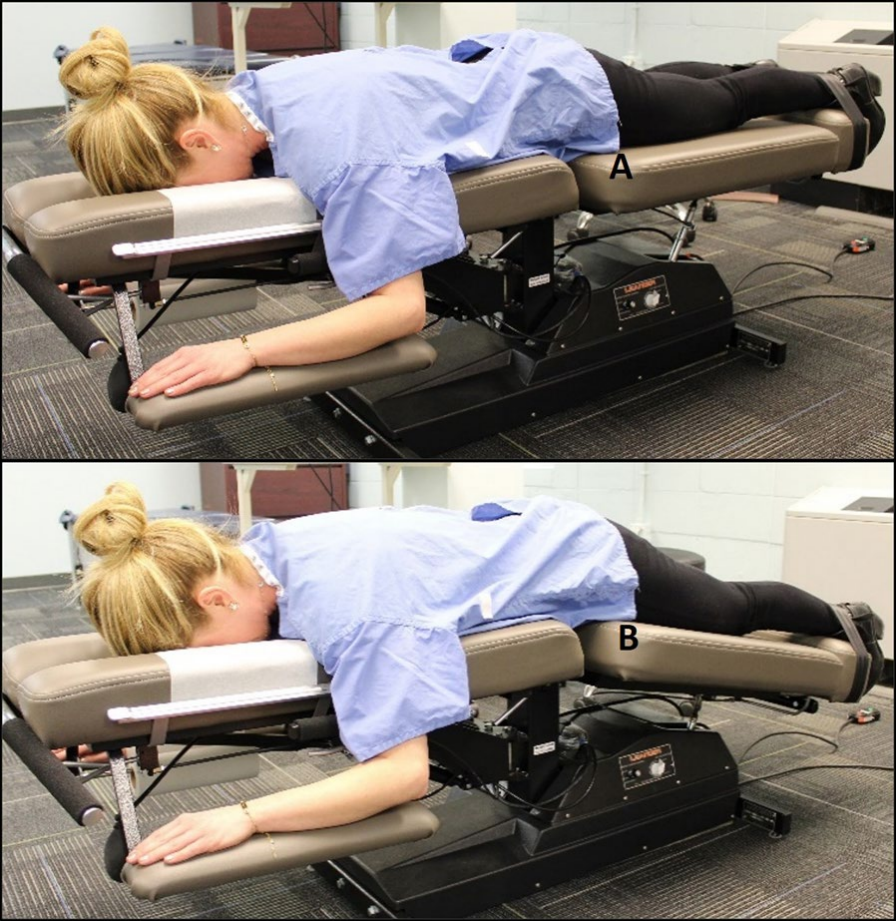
Participant positioning for ultrasound imaging. An automated table slowly moves the lower extremities from a neutral position (A), 15 degrees downward B and back to neutral, cycling 5 times over 10s
